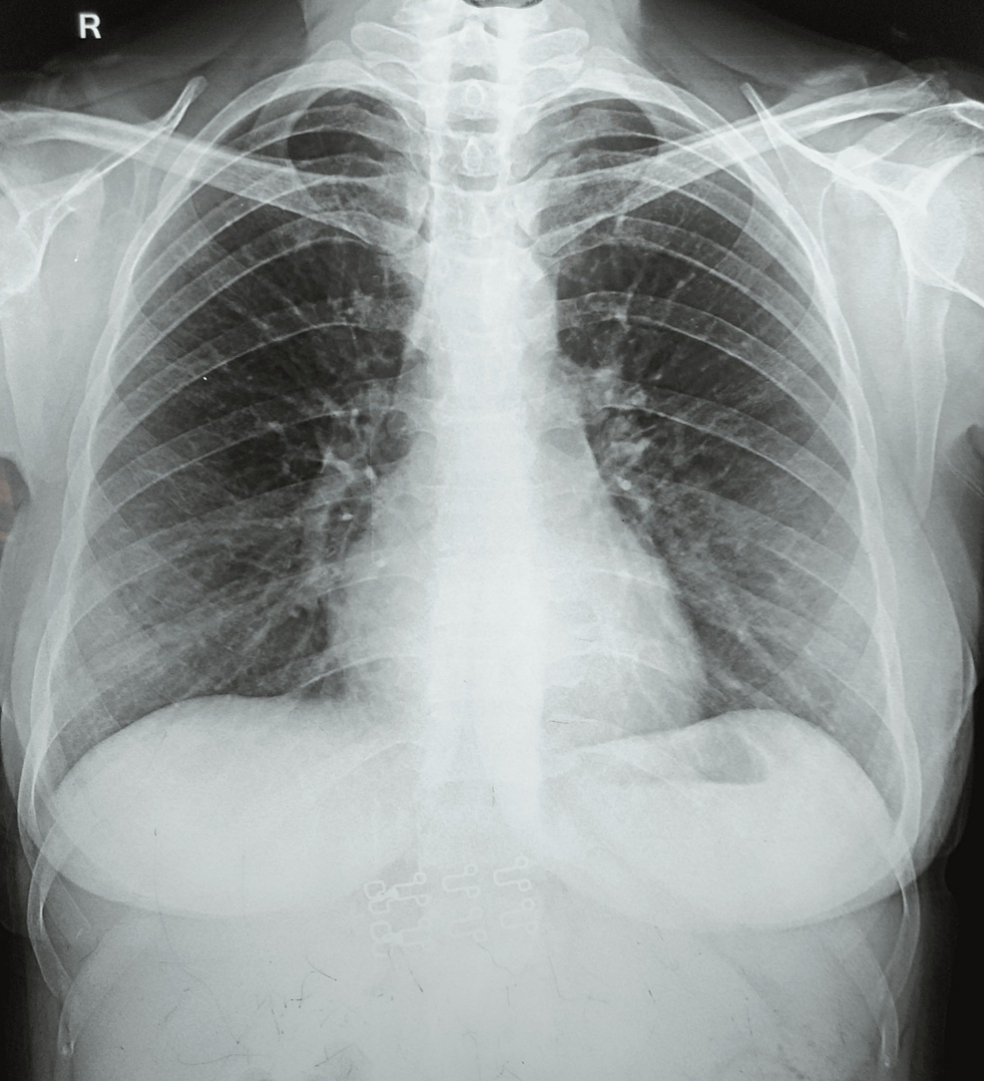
A normal chest radiograph.
